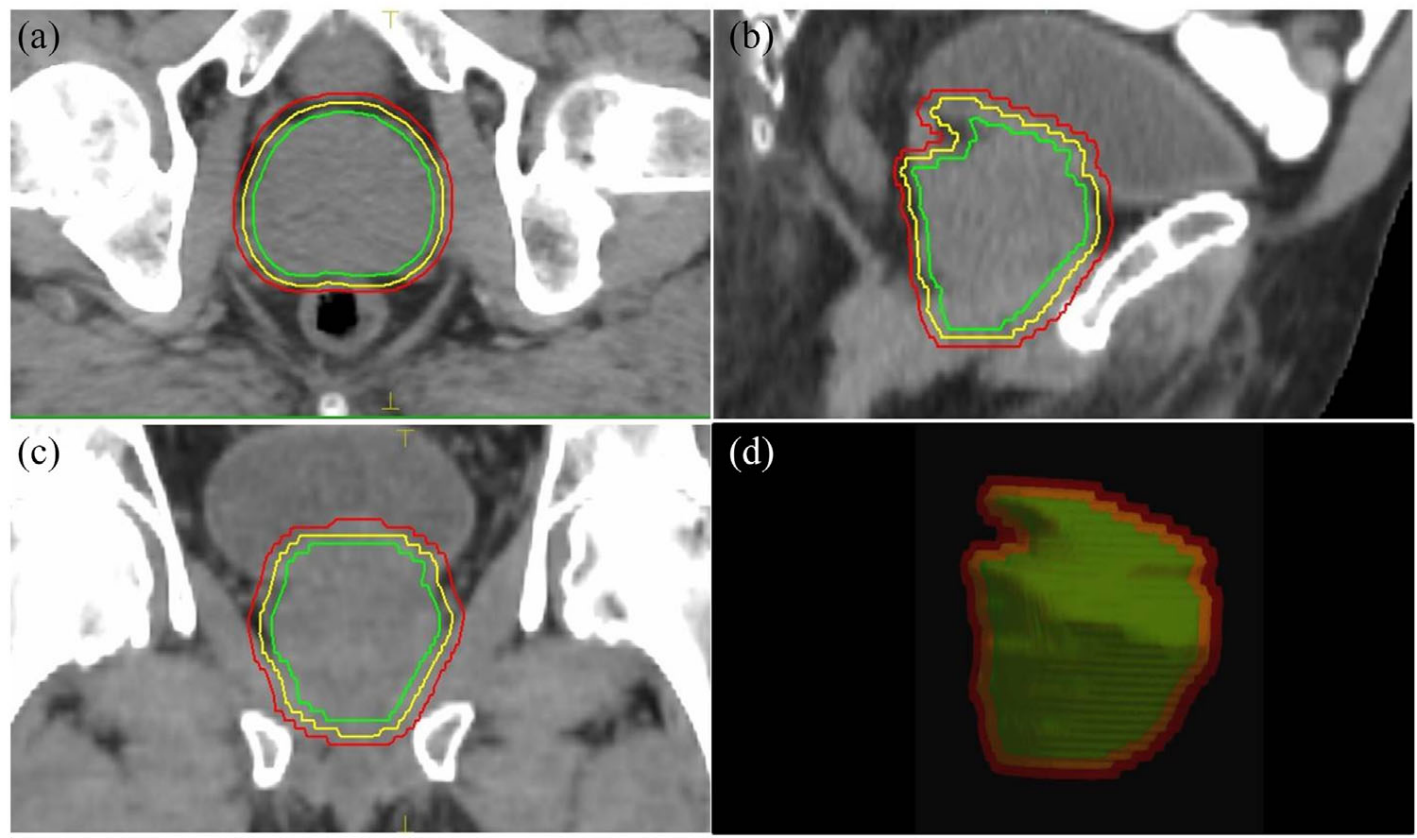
Prostate (green line), PTV with reduced margin (yellow line) and PTV with standard margins demonstrated in (a) axial, (b) sagittal, (c) coronal CT slices, and (d) beams‐eye‐view reconstruction.
